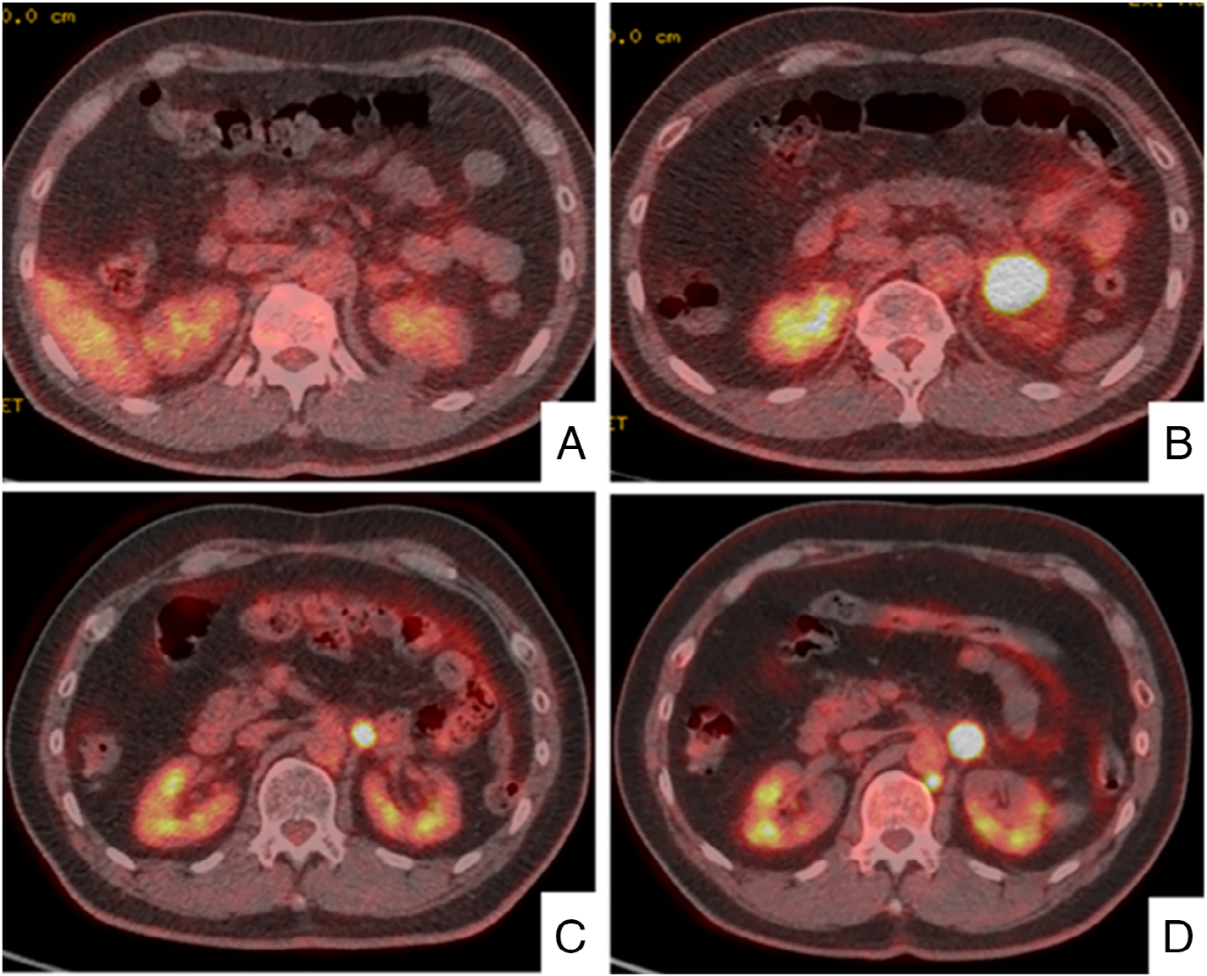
18F‐fluorodeoxyglucose positron emission tomography (18F‐FDG PET) before and after adrenalectomy. There is no sign of adrenal metastasis after thoracoscopic upper right lobectomy (A). Eleven months after surgery, an enlargement of the left adrenal gland appeared (B). Eight months after adrenalectomy, a follow‐up 18F‐FDG PET scan showed uptake in an enlarged lymph node near the resected region (C). Forty‐one months after adrenalectomy, clear enlargement of the lesion was seen (D).
